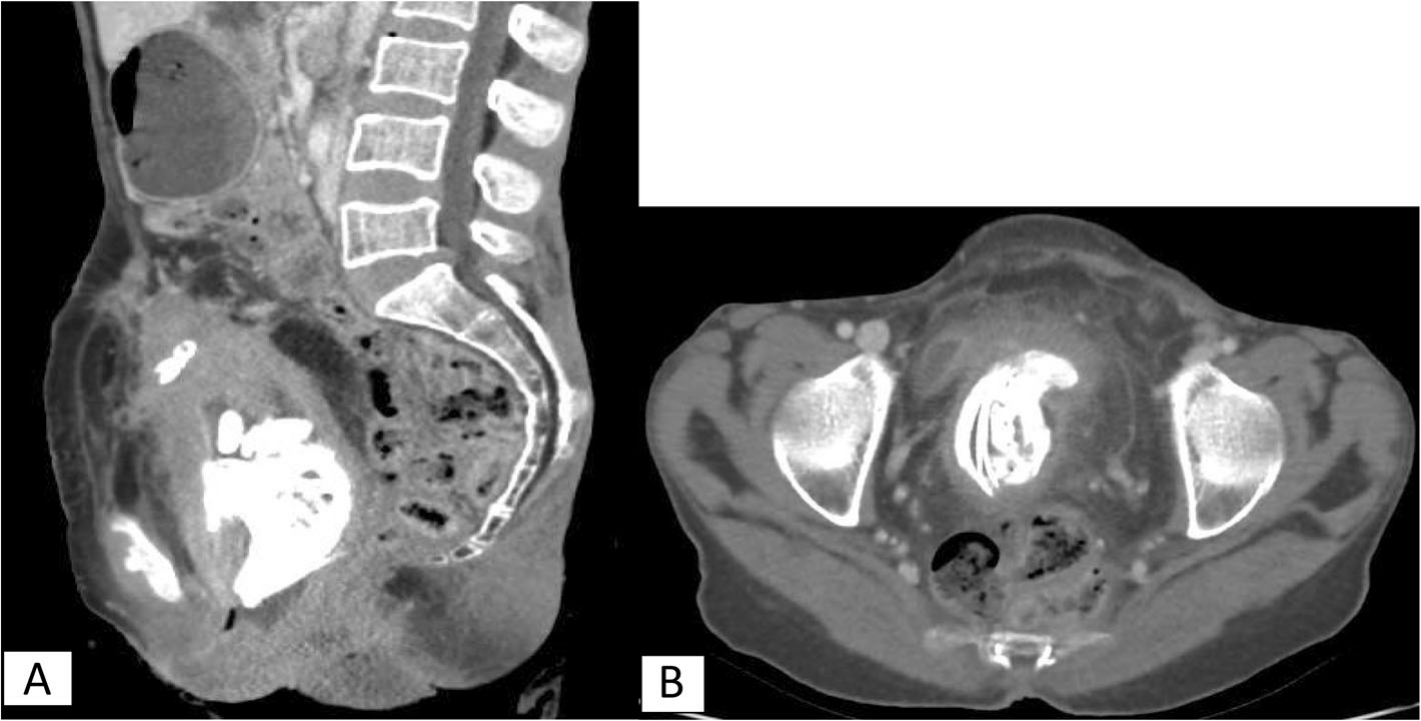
Post-contrast abdominal computed tomography images. a Sagittal image showing intrauterine fetal bony parts and focal adhesion of the uterus to the anterior abdominal wall. b. Axial image showing multiple bony structures of the fetus and displaced urinary bladder anterolateral
